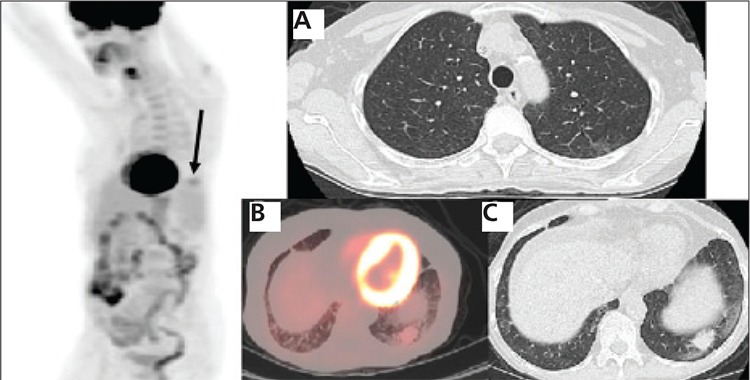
Two months after Rituxan therapy a restagingPET/CT shows a faint focus of abnormal increased FDG uptake inthe left lower lateral chest (arrow) and complete resolution of theFDG uptake in the upper medial chest on the 3D PET image. On theaxial CT image (A) the upper lobe lesion has completely resolved. Thefused axial image (B) and axial CT slice (C) show interval decrease inthe size and FDG uptake of the lower lobe lesion, to 2.4x1.7 cm withSUV max of 2.4, representing favorable response to treatment
